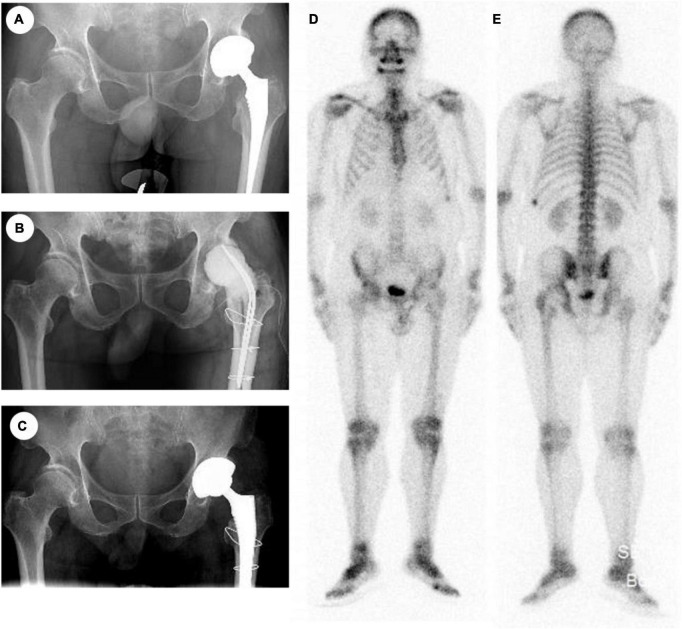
(A) X-ray of primary operation. (B) X-ray of two-stage reconstruction. (C) X-ray after revision. (D,E) Bone scan.
